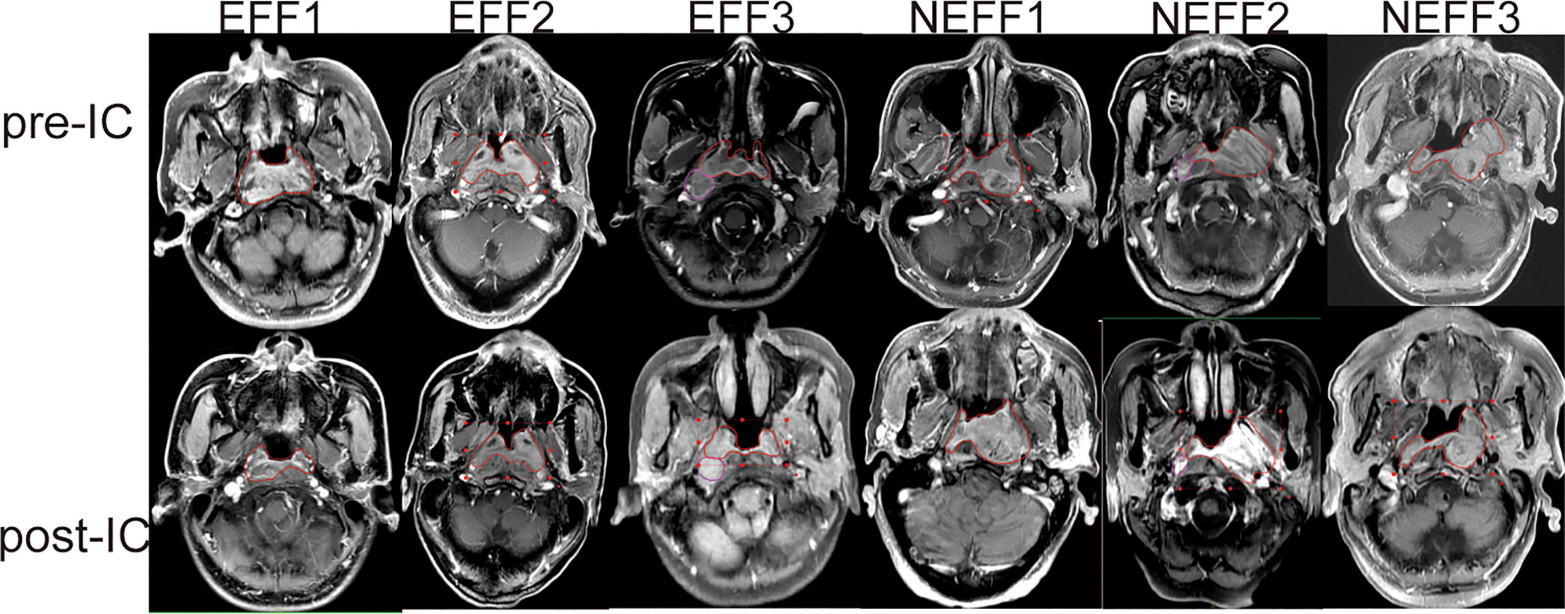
Typical MRI images for GTV changes before and after IC. MRI images of three patients with PR (EFF1-3), one patient with PD (NEFF1), and two patients with SD (NEFF2-3) at baseline (pre-IC) and after two cycles of IC (post-IC). The area within the red line is the primary focus. MRI, magnetic resonance imaging; GTV, gross tumour volume; IC, induction chemotherapy; EFF, effective; NEFF, non-effective.
